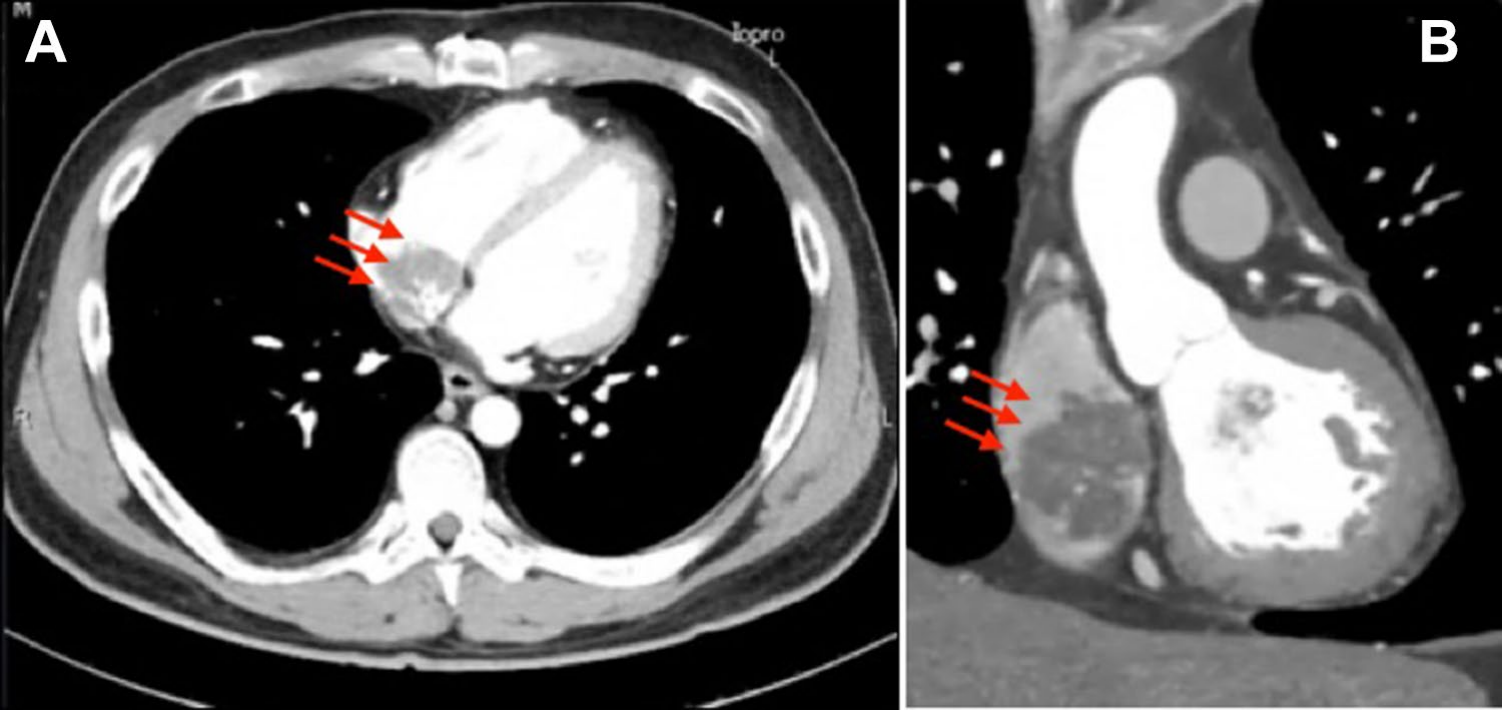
Preoperative computed tomography images showing a 32 × 26-mm-diameter tumor attached to the atrial septum (red arrow) a Axial view b Coronal view
